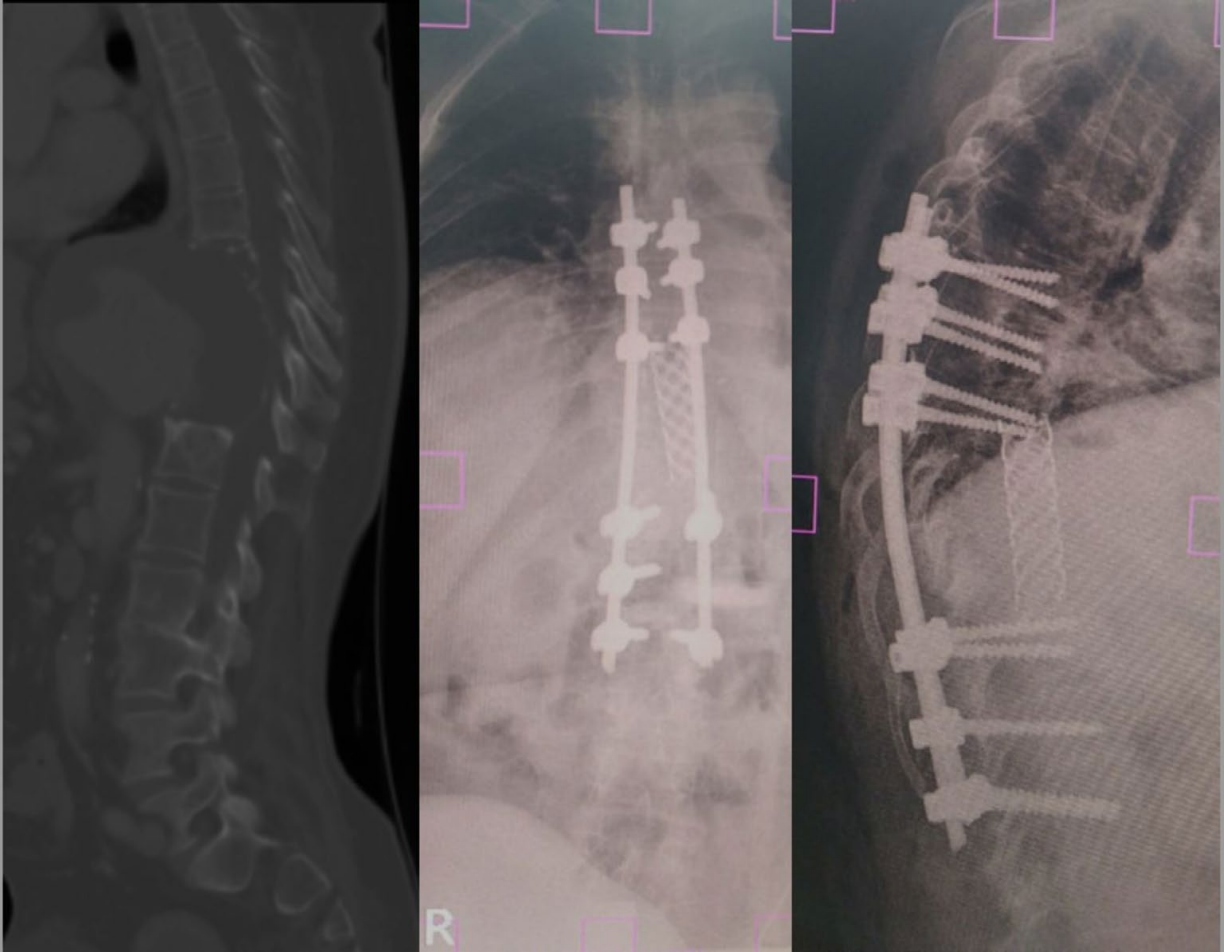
Spinal reconstruction images. (A) Preoperative sagittal CT showing severe anterior vertebral body erosion and kyphotic deformity centered at T9–T11. (B) Postoperative axial CT demonstrating central placement of the interbody cage within the resected vertebral space. (C) Postoperative sagittal CT or X‐ray showing correction of kyphosis and posterior instrumentation spanning T6 to L2 with pedicle screws and rods.
